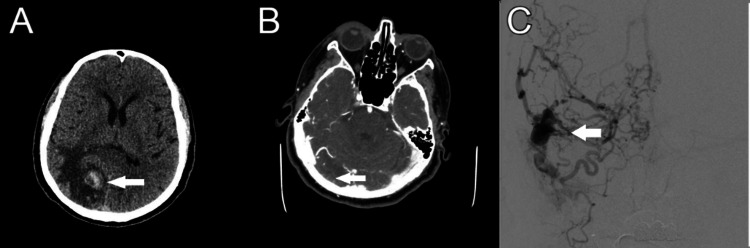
Selected initial neuroimaging A) Computed tomography head demonstrating right occipital hemorrhage with vasogenic edema. B) Computed tomography and angiography demonstrate a filling defect in the medial right transverse sinus and prominent cortical veins, which could be suggestive of underlying DAVF. C) Digital subtraction angiography demonstrating right transverse dAVF with cortical venous drainage.
